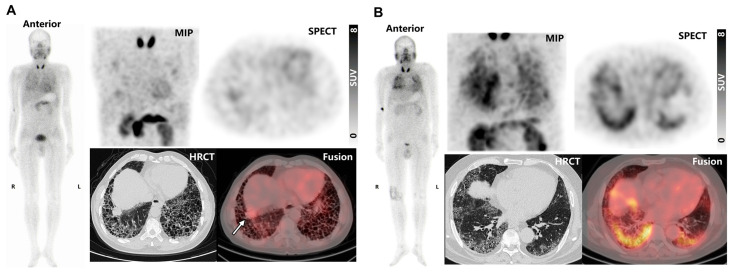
(A) ^99m^Tc-H-PoFP_2_ SPECT/CT and HRCT images of a 65-year-old male patient. The solid lesion in the right basal part was noted by a white arrow. (B) ^99m^Tc-H-PoFP_2_ SPECT/CT and HRCT images of a 63-year-old male patient. MIP, maximum intensity projection.
